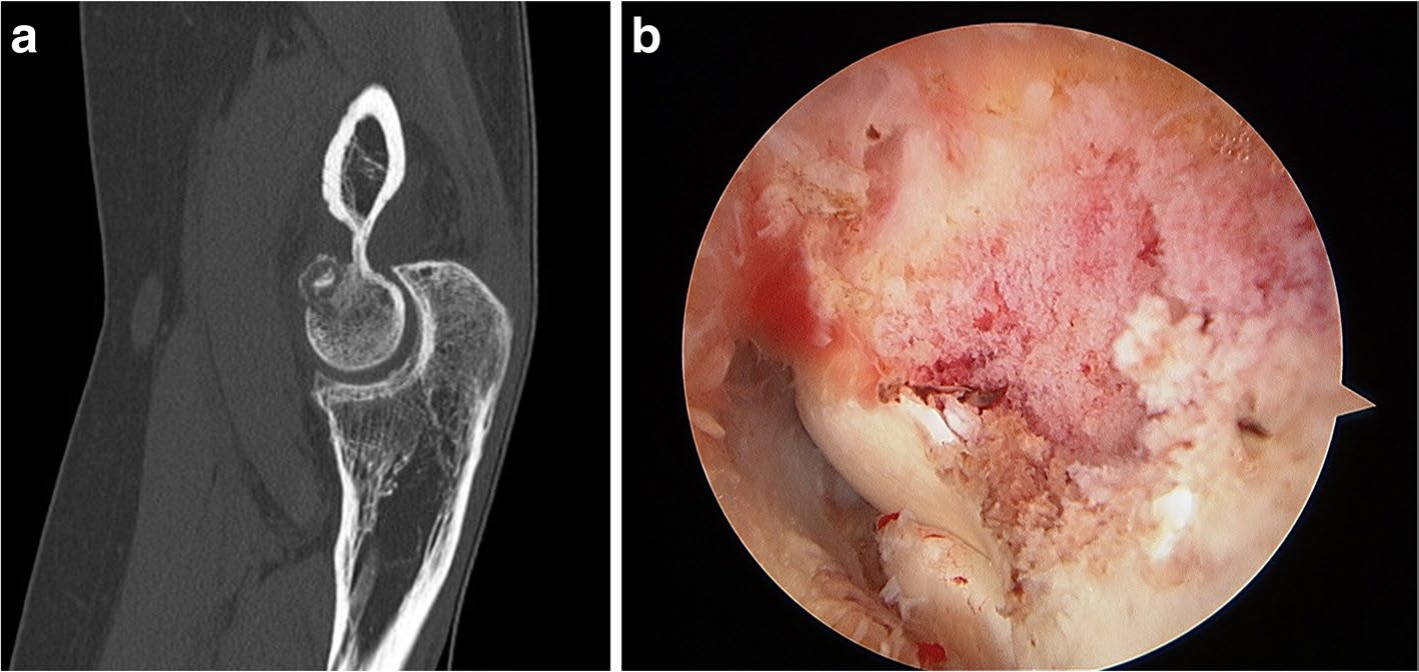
Arthroscopic removal of an osteoid osteoma. A Preoperative CT scan, B Arthroscopic view of the nidus prior to resection
